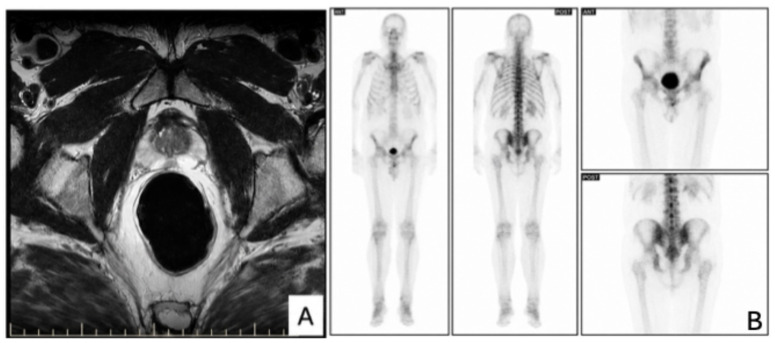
Preoperative imaging evaluation. (A) Axial T2-weighted multiparametric MRI of the prostate demonstrating a focal hypointense lesion in the peripheral zone, suspicious for clinically significant prostate cancer (PI-RADS 4–5). Diffusion-weighted imaging (DWI) with corresponding apparent diffusion coefficient (ADC) reduction supported restricted diffusion. (B) Whole-body bone scintigraphy showing no evidence of skeletal metastatic disease.
